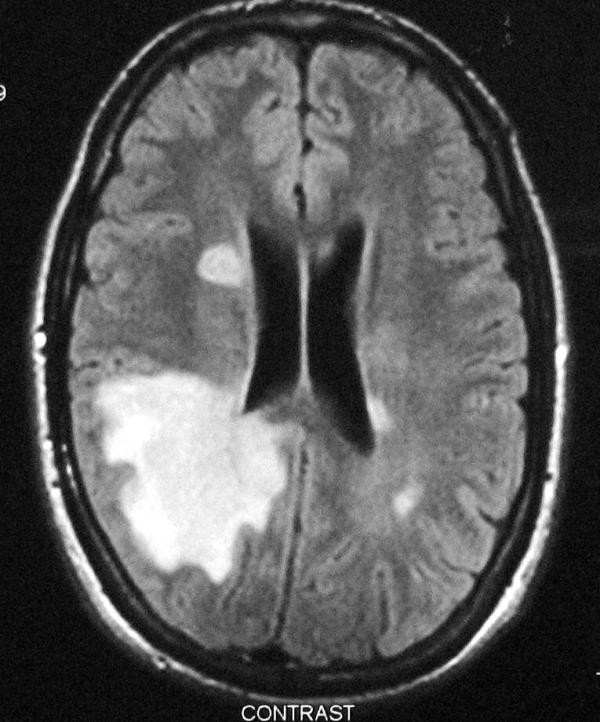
MRI upon admission: T2W & Flair sequences revealed a lesion extending from the right parietal to the temporal lobe, compressing the posterior aspect of the right ventricle. No lesion showed gadolinium enhancement.
